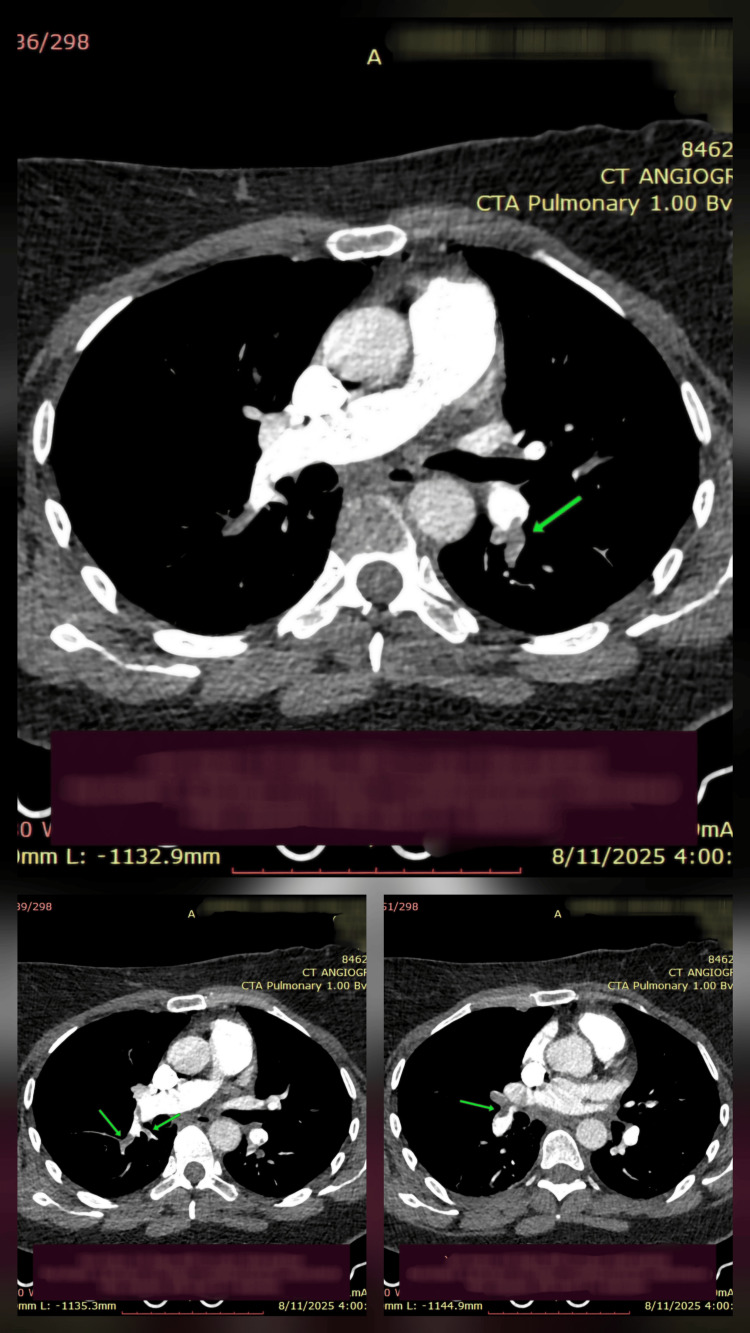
Computed tomography angiography axial image demonstrating pulmonary embolism (arrows).
